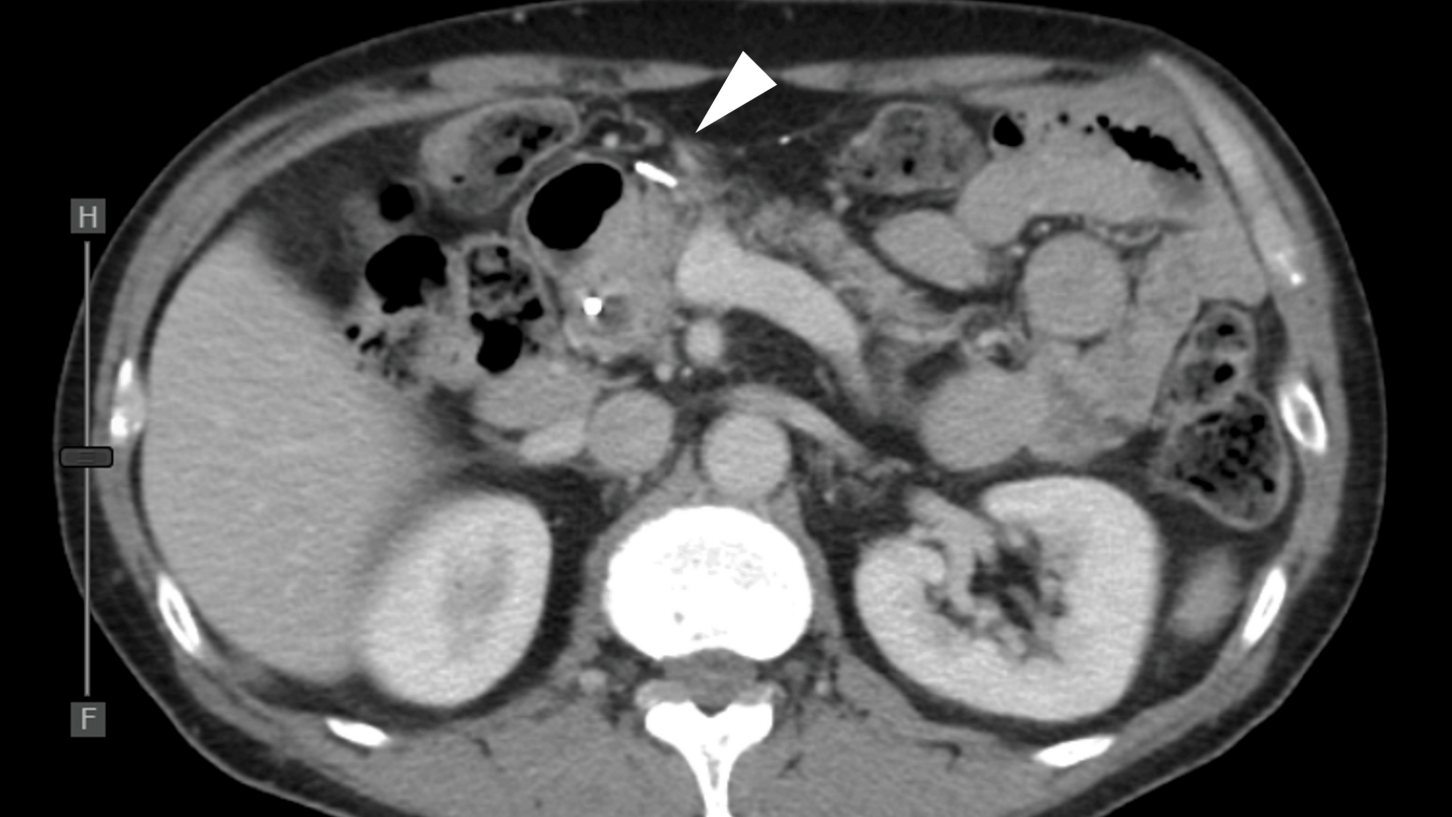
Axial imaging of abdominal contrast-enhanced computed tomography eight months after immune-related sclerosing cholangitis No re-enlargement of the lymph nodes is observed (arrowhead).
